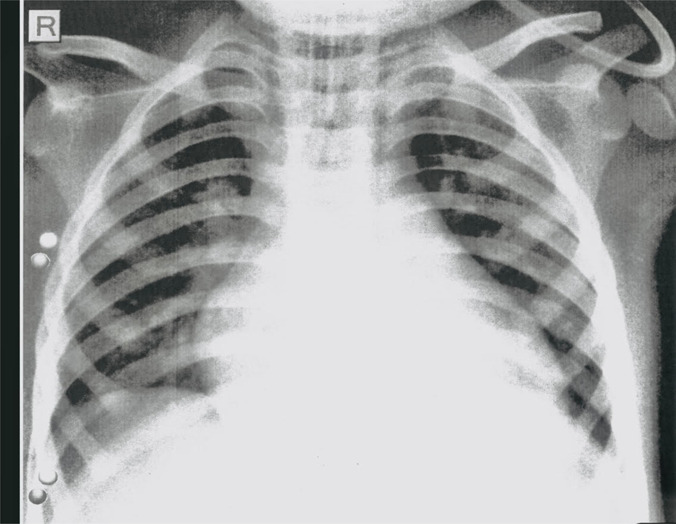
Chest X-ray: Cardiomegaly with bilateral plethoric lung fields
